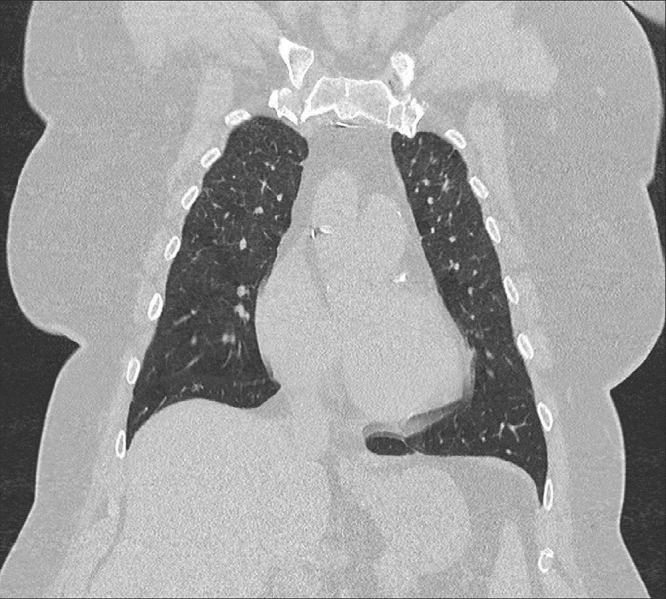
Computed tomography of the chest repeated after avoidance of hot tub and treatment with systemic steroids demonstrating significant improvement
